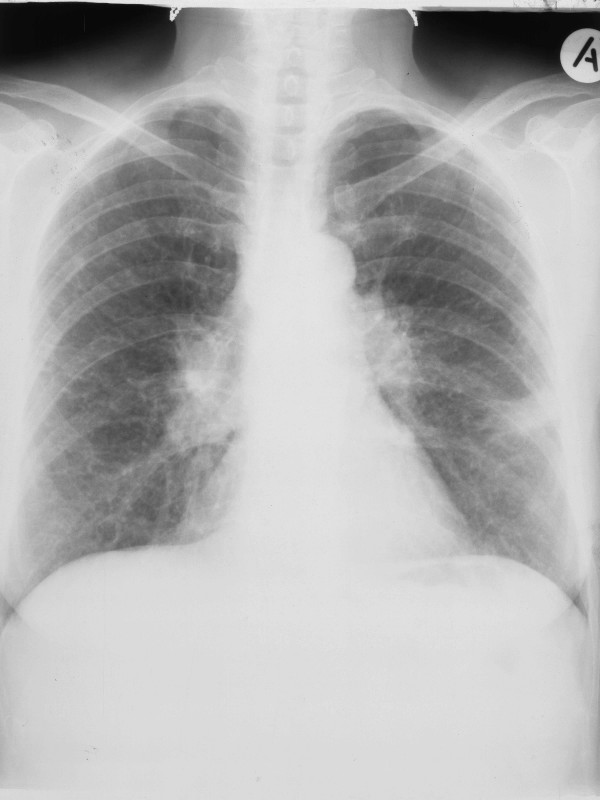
Chest X-ray showing extended bilateral interstitial lung densities and left lower lung field consolidation.
